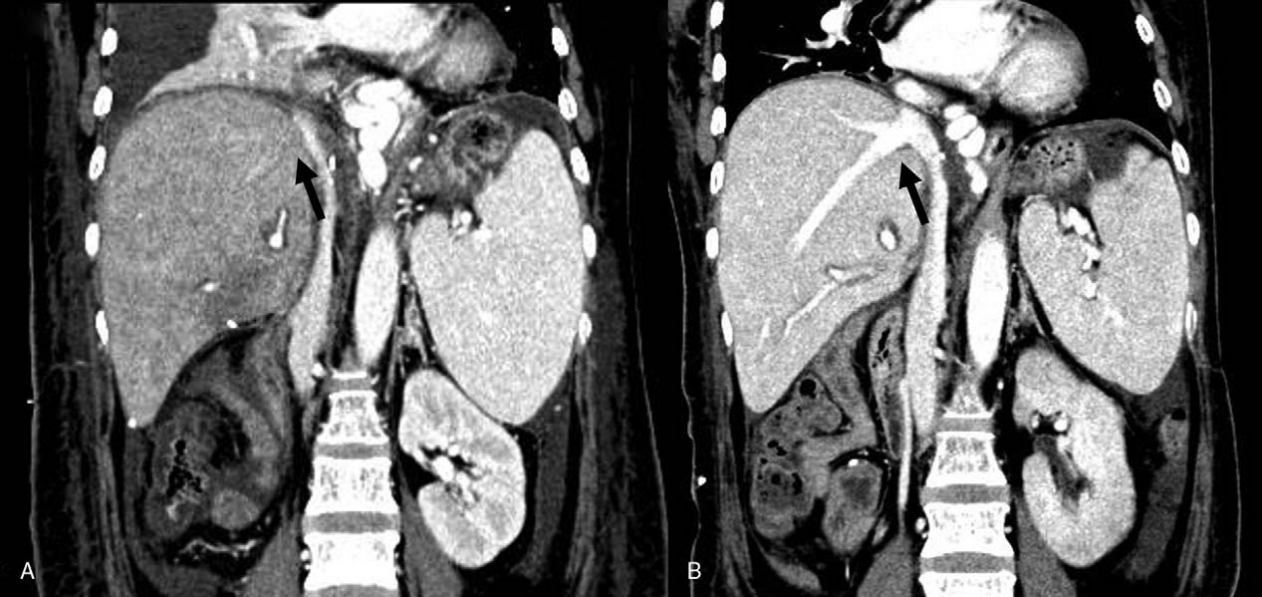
(A) Abdominal computed tomography image on postoperative day 14 showing heterogeneous enhancement of the liver graft with massive pleural effusion and ascites. The right hepatic vein (arrow) is obscured. (B) Abdominal computed tomography on postoperative day 43 showing improvement of the veno-occlusive disease. Pleural effusion and ascites are markedly decreased. The enhanced pattern of the liver graft is homogeneous, and the right hepatic vein (arrow) reappears.
